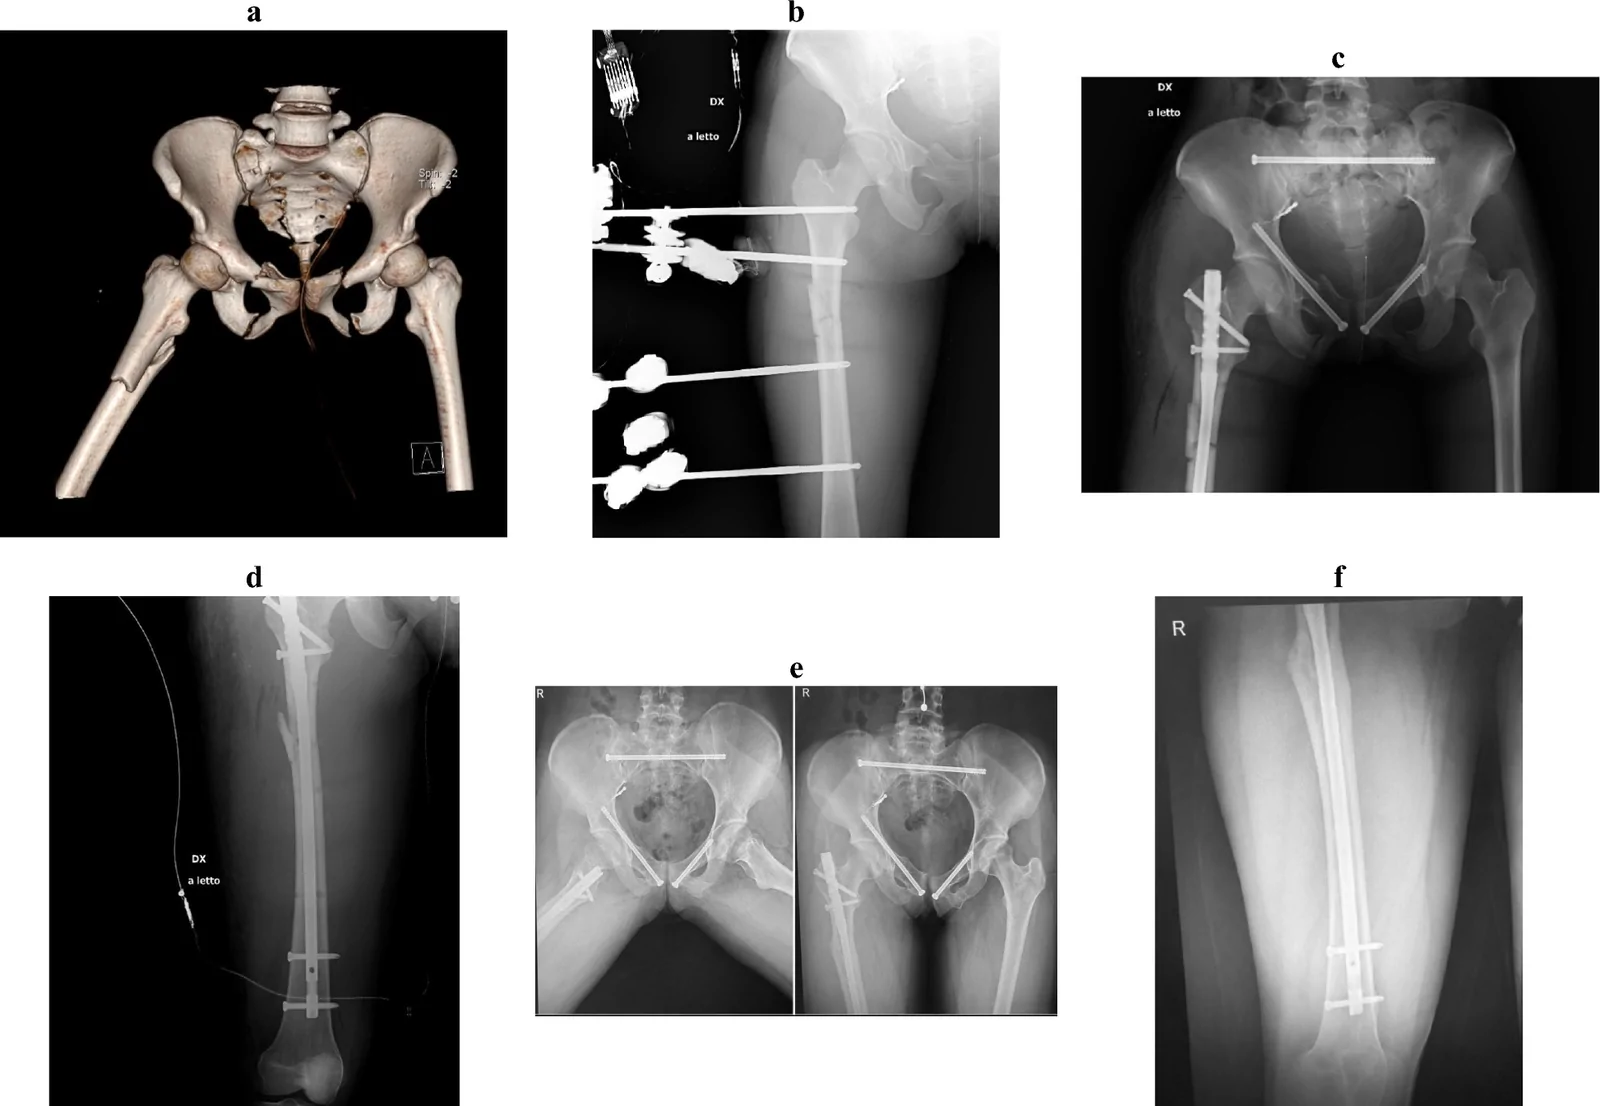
Representative case of a 19-year-old woman with a high-energy AO/OTA 32-B2 femoral shaft fracture associated with an unstable pelvic ring injury, managed with a Bactiguard-coated intramedullary nail. (a) Preoperative three-dimensional computed tomography reconstruction demonstrating the femoral and pelvic injuries. (b) Temporary damage-control external fixation of the femur. (c, d) Immediate postoperative anteroposterior and lateral radiographs after definitive fixation with a Bactiguard-coated intramedullary nail and concomitant pelvic stabilisation. (e, f) Radiographs obtained at the 18-month follow-up, demonstrating fracture union and maintained alignment without implant-related complications
